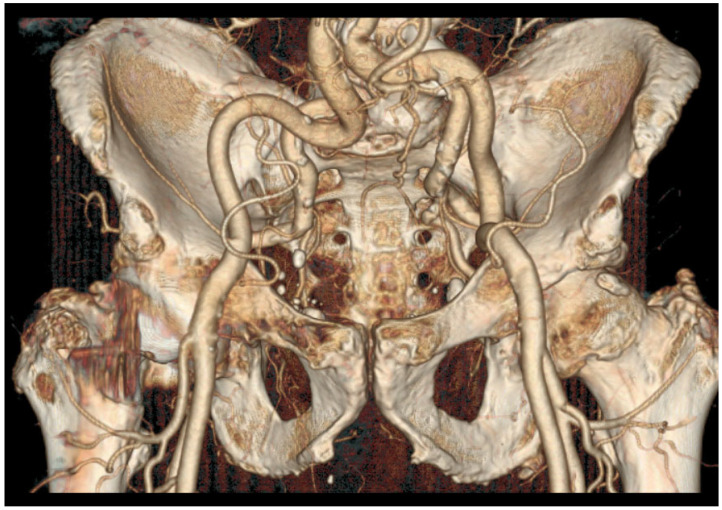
A CT scan with a 3D reconstruction showing very tortuous iliac arteries and very acute right internal/external iliac angulation. Upper radial access was privileged in the first intention.
